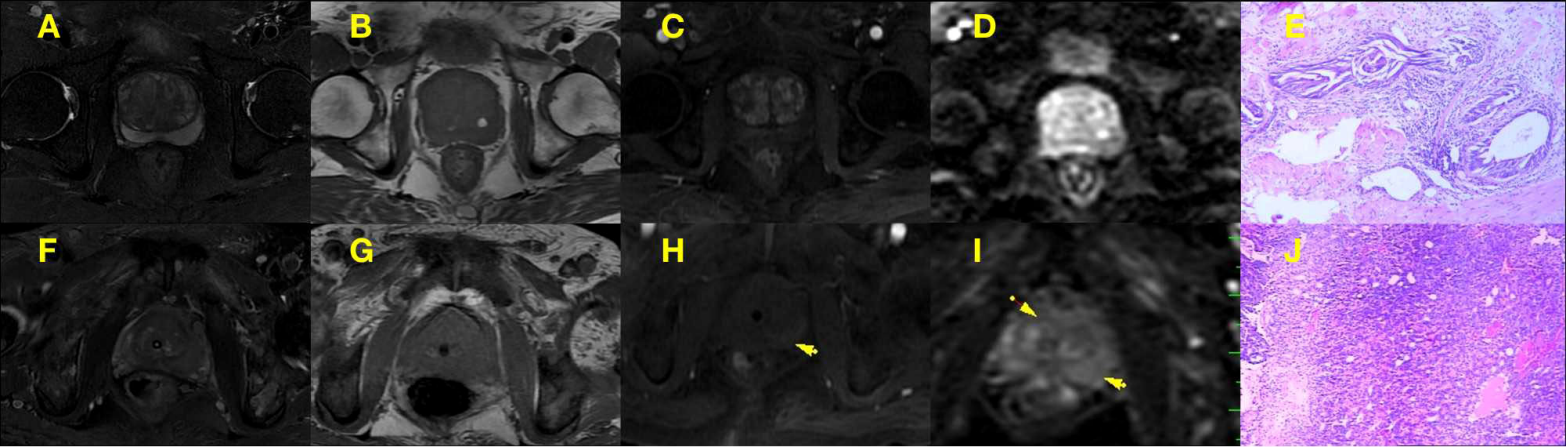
(A–E) PI-RADS 2 scored alterations. An example of PI-RADS 2 lesion confirmed at biopsy as pathologic examination of BPH patient (E), multi-parameter MRI showed hypoint T2 signal intensity in the peripheral zone, iso and hyper T2 signal intensity in the central gland(A), iso and hyper T1 signal intensity in the central gland (B), normal diffusivity at b800 DWI (C) with contrast enhancement (D), that the peripheral zone in the multi parameter MRI showed 2 points and the central gland in the multi parameter MRI showed 2 points, DWI showed 1 points. (F–J) PI-RADS 5 scored alterations. An example of PI-RADS 5 lesion confirmed at biopsy as pathologic examination of PCa patient (J), multi-parameter MRI showed so and hyper T2 signal intensity in the central gland and peripheral zone (F), iso and hyper T1 signal intensity in the central gland (G), hyperintensity signal at b800 DWI (H) with contrast enhancement (I), that the T2WI showed 5 points and DWI showed 5 points.
